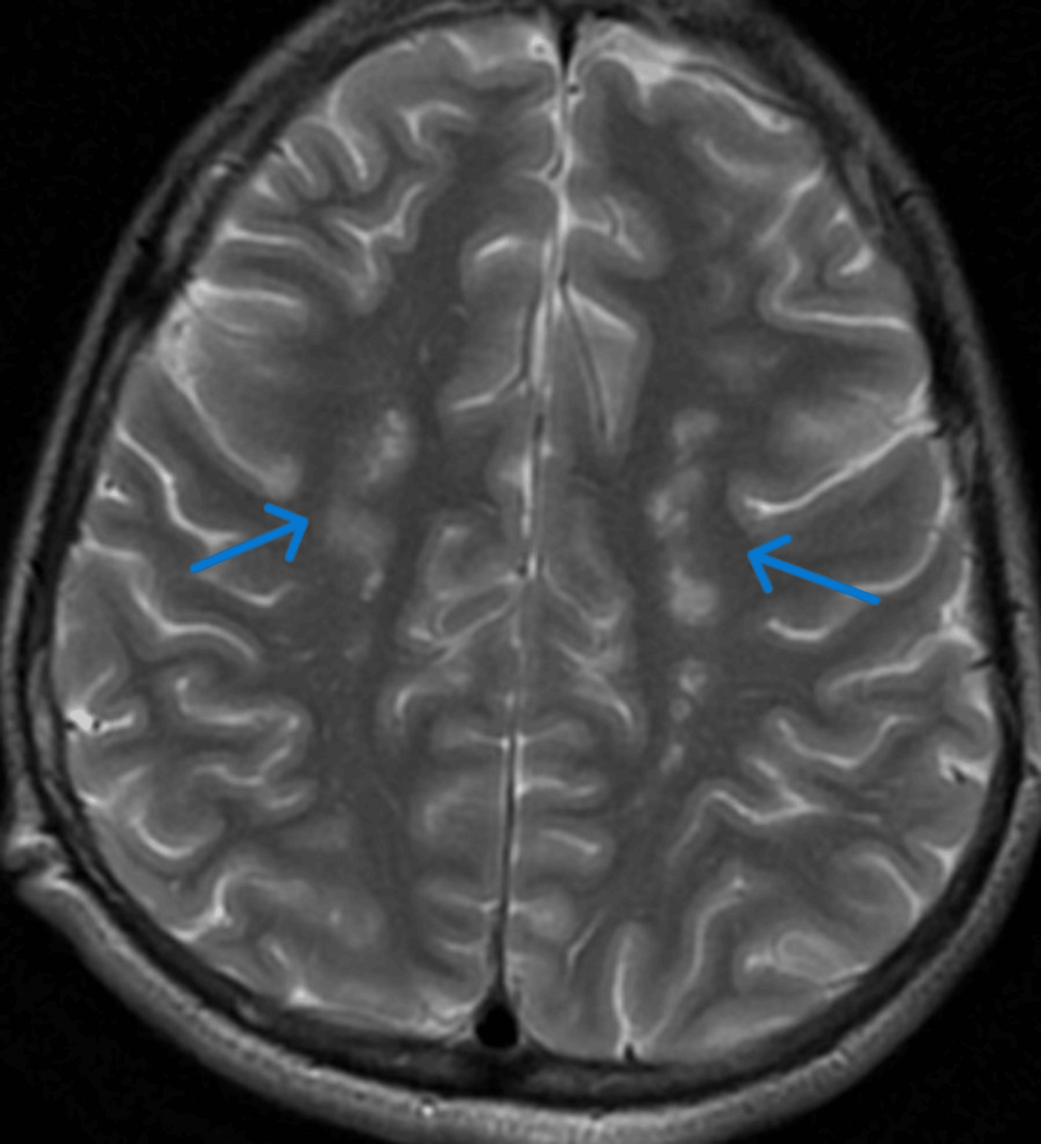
Contrast-enhanced T2-weighted MRI brain film showing parieto-temporal hyperintensities
